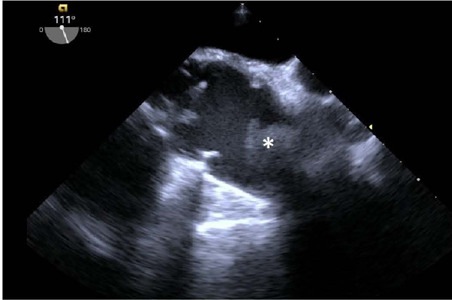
Transesophageal echocardiography showing the mass (*) in the ascending
